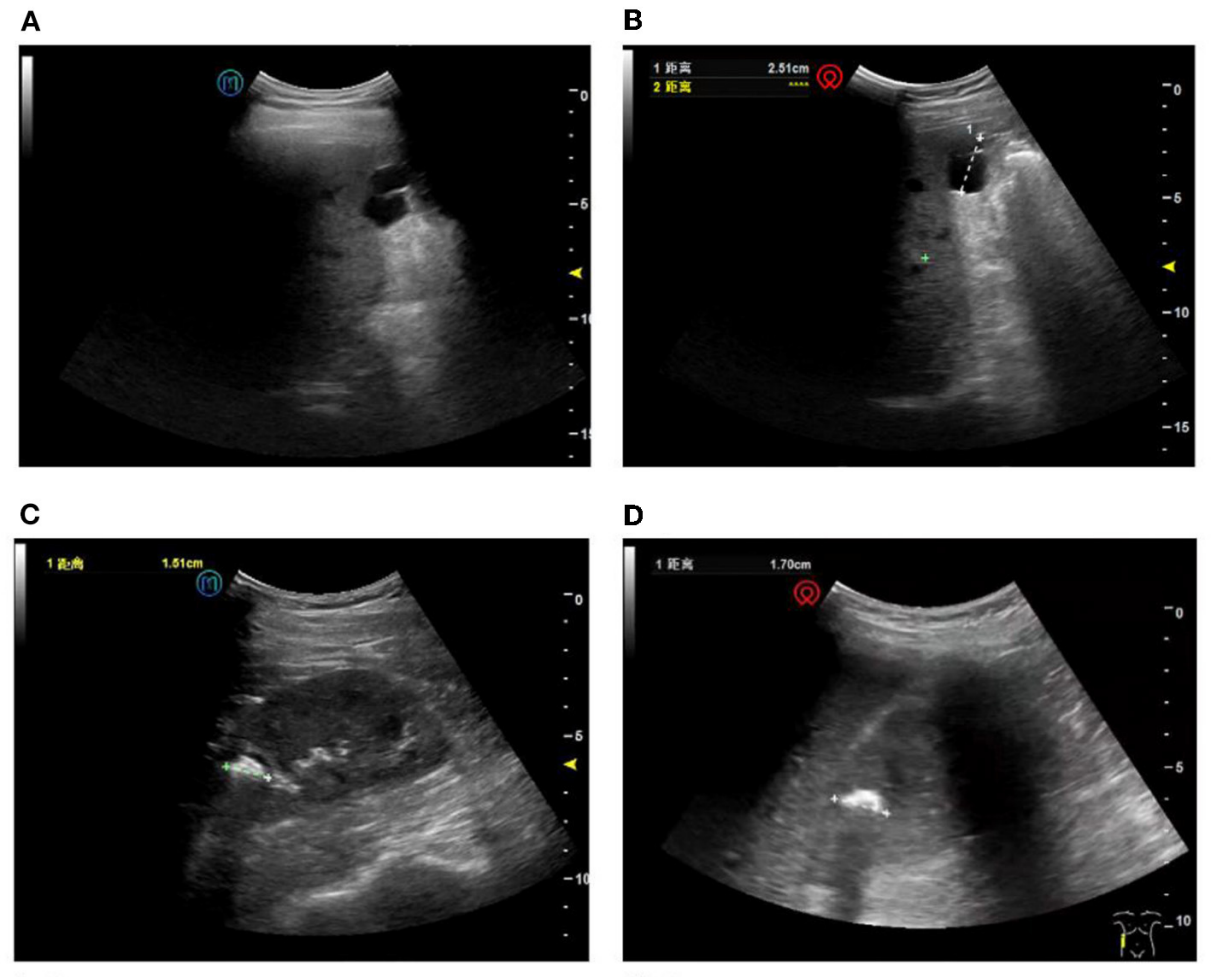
Comparison of the results of the two ultrasound examinations. (A) Remote ultrasound image showing a liver cyst. (B) Confirmation of the liver cyst using bedside ultrasound. (C) Remote ultrasound image showing a right kidney stone. (D) Confirmation of the lesion using bedside ultrasound.
